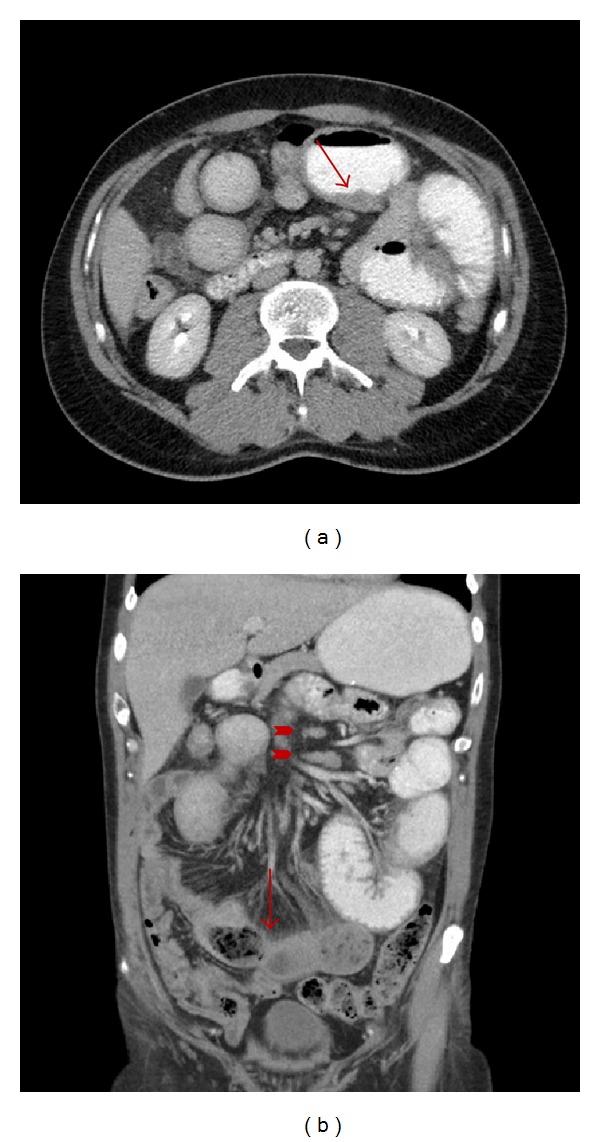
Axial (a) and coronal (b) CT images obtained after IV and oral contrast administration are shown. Note asymmetric wall thickening involving some of the ileal bowel loops (arrow in (a)). There is dilation of several small bowel loops with a transition point in the mid-lower quadrant (arrow in (b)) consistent with small bowel obstruction. There are also a few borderline enlarged mesenteric lymph nodes (arrowheads in (b)).
